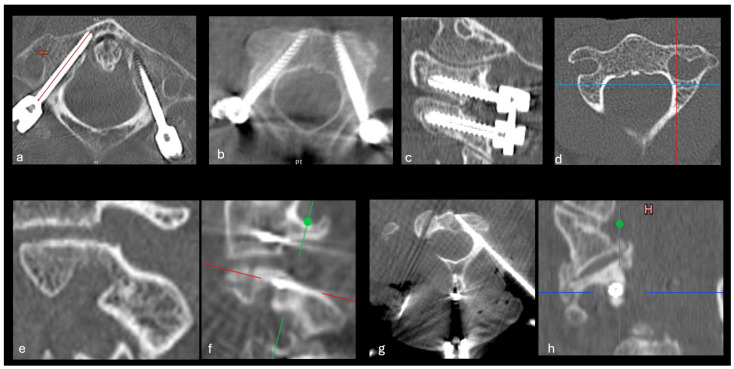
Clinical Imaging and grading analysis. (a–c) Intraoperative CT assessment (C1–C2): post-placement view of C1 and C2 screws following a posterolateral trajectory. The placement is classified as Gertzbein–Robbins Grade A (the screw is entirely contained within the pedicle/vertebral body with no cortical breach) and Heary Grade I (the screw tip is contained within the bone and does not violate the anterior vertebral wall). (d,e) Pedicle with high-riding vertebral artery: sagittal and axial views of a C2 pedicle in a patient with a high-riding vertebral artery. (f,g) K-wire trajectory HRVA: in these instances, the trajectory is adjusted to be more medially oriented and cephalad. This modified approach maximizes cortical bone purchase while ensuring that the screw remains safely clear of the vertebral artery’s path. (h) Intraoperative coronal view confirming successful screw placement within a narrow pedicle in the presence of an HRVA. The screw is positioned outside the transverse foramen, maintaining cortical integrity without violating the vessel.
